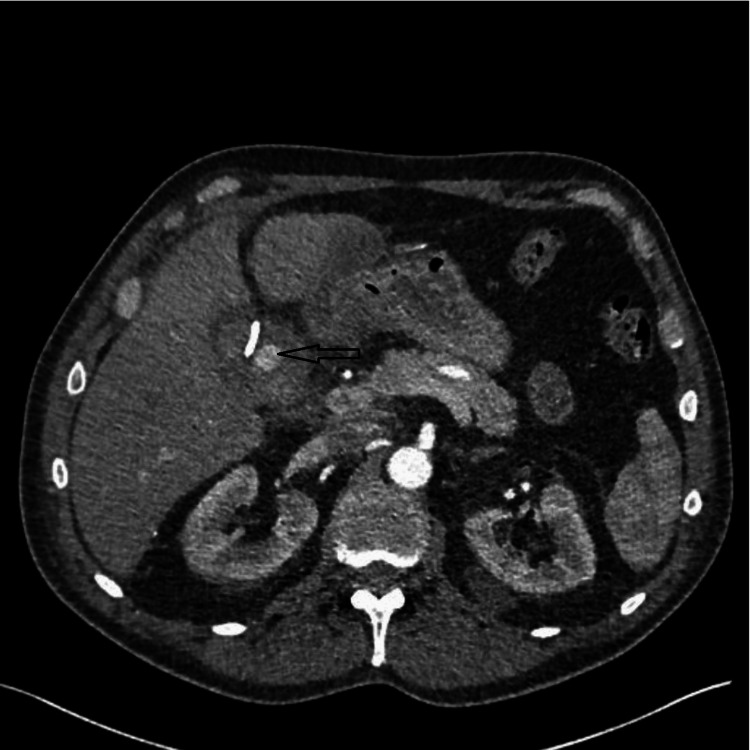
CT angiogram showing a pseudoaneurysm of the cystic artery within the gallbladder (see arrow)
